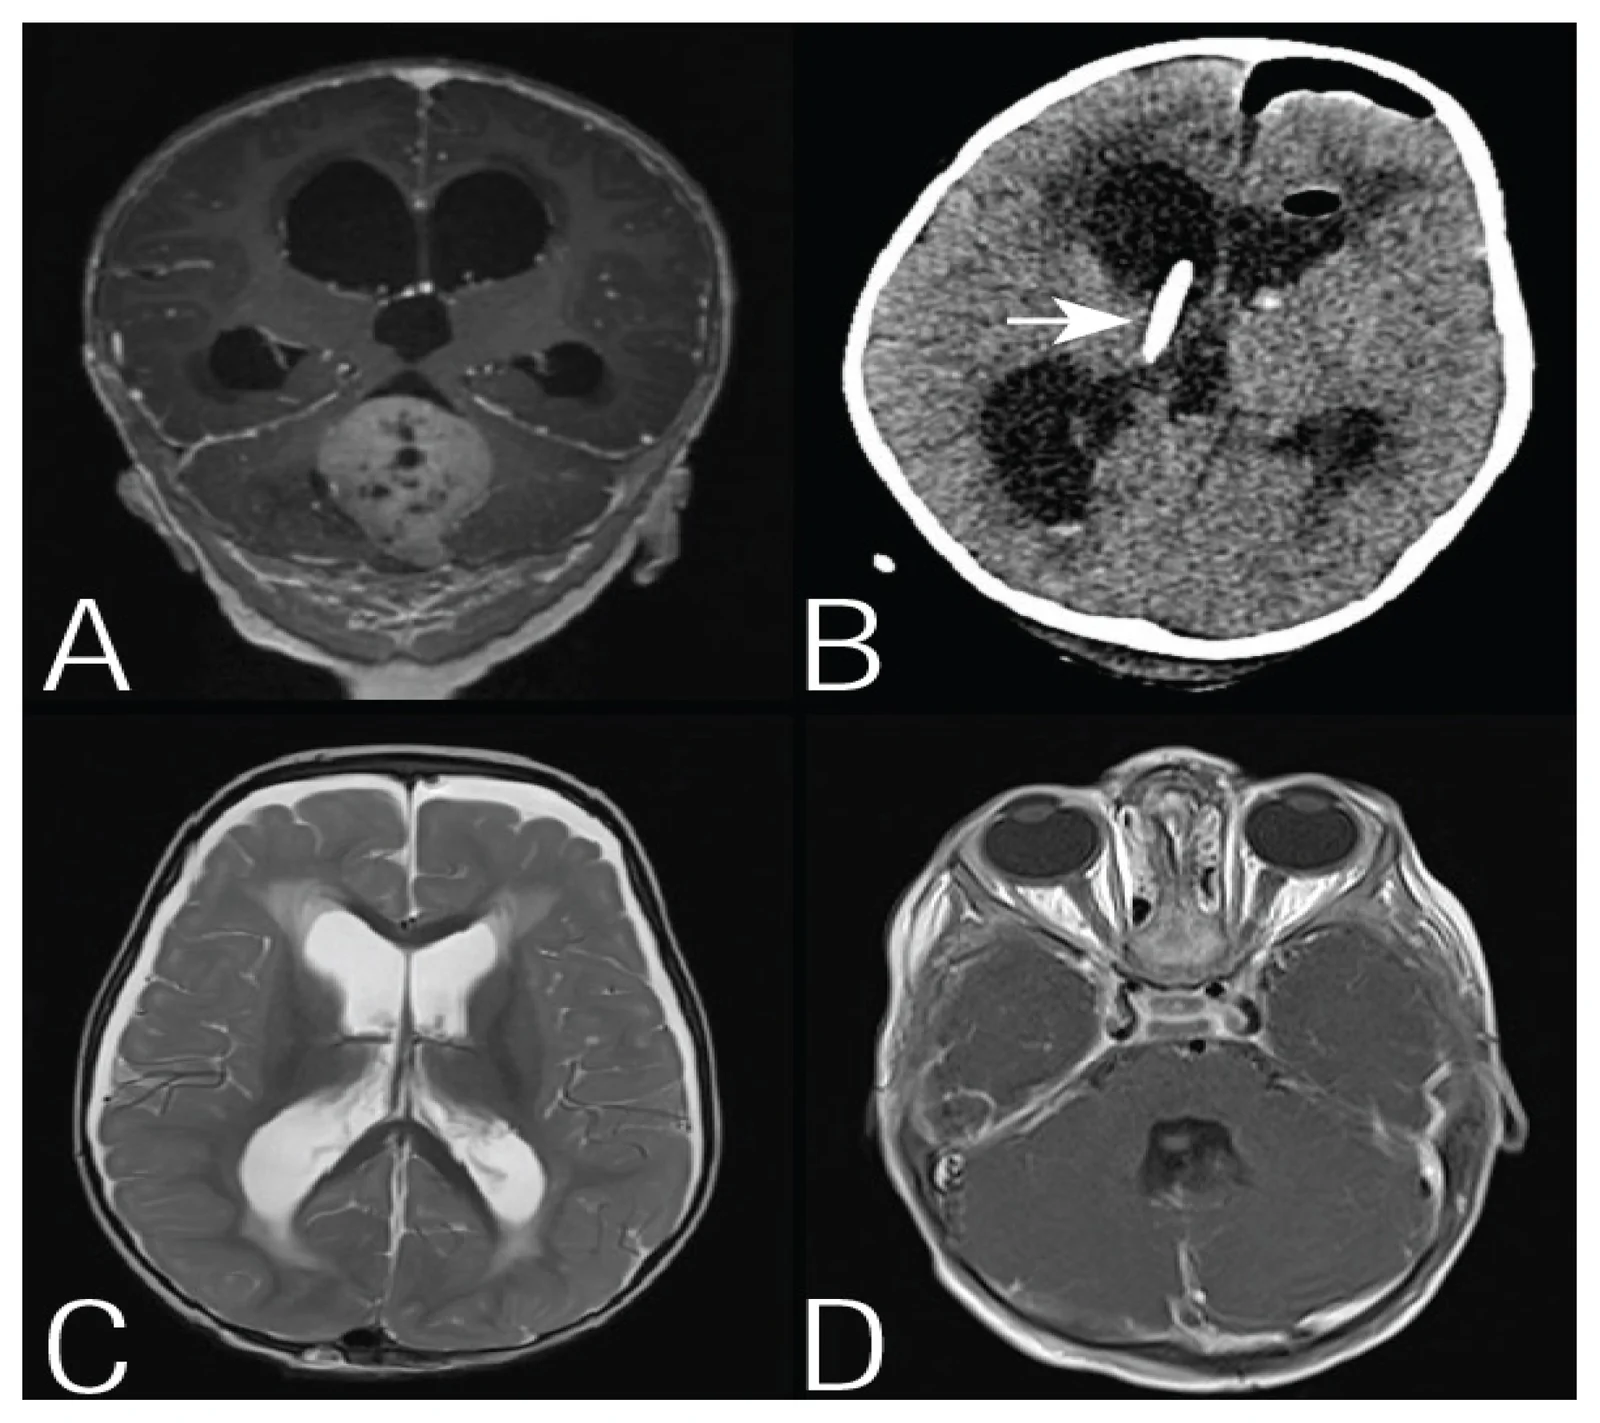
A: A coronal T1W, gadolinium enhanced magnetic resonance imaging (MRI) scan of the brain showing midline posterior fossa tumour with hydrocephalus. B: An axial section of a postoperative computed tomography scan 24 hours after clamping the external ventricular drain showing persistent hydrocephalus and ventricular catheter in the right lateral ventricle (arrow). C: An axial T2W postoperative MRI of the brain 72 hours after removal of external ventricular drain showing thin subdural hygroma and regression of hydrocephalus. D: An axial T1W, gadolinium enhanced MRI showing no residual tumour.
